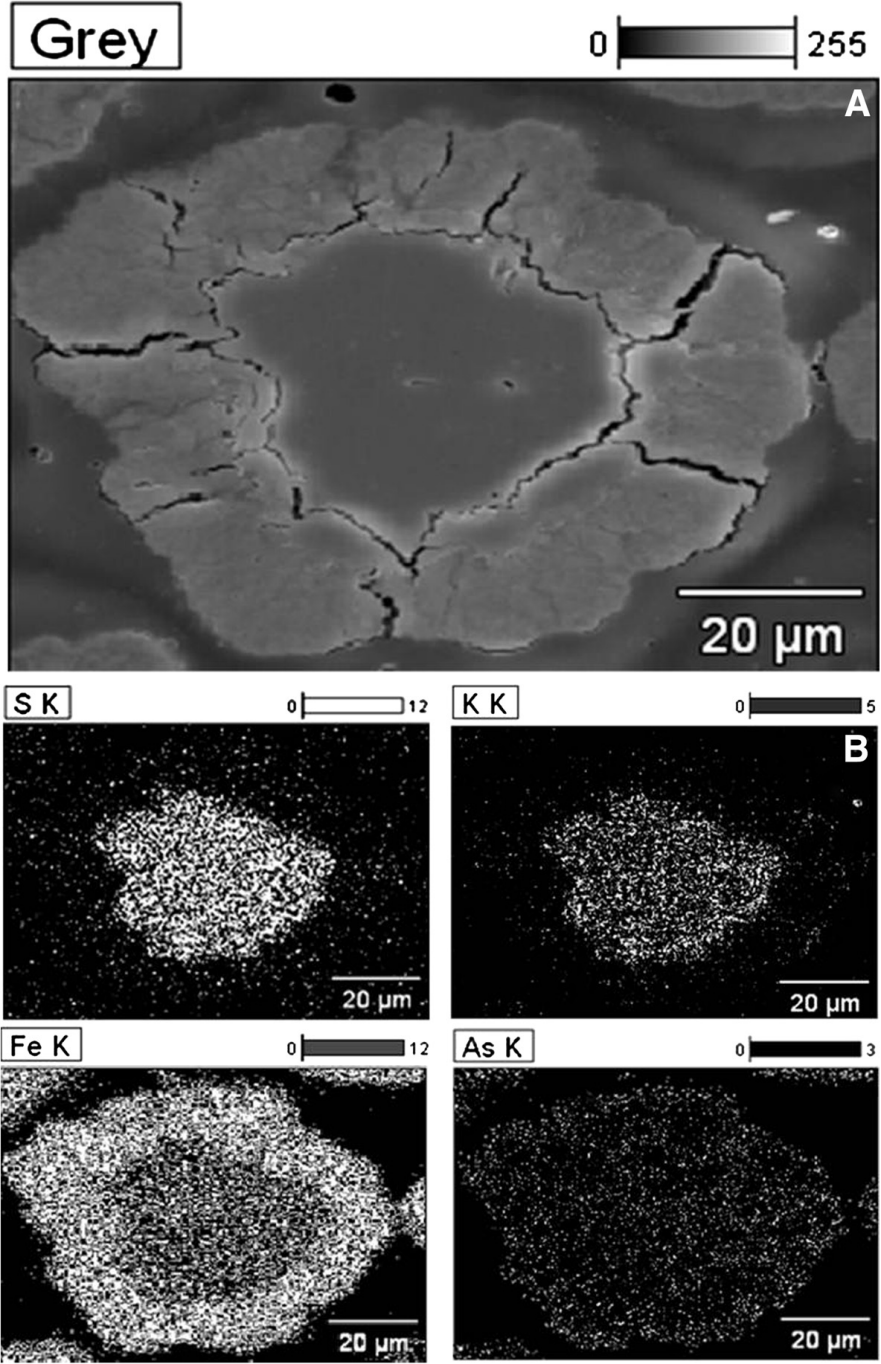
(A) Partially reacted particle and (B) X-ray map. Figure 5A shows a partially decomposed particle made of a gel halo, a reaction front and an unreacting core. Figure 5B shows the mapping performed on the partially decomposed particle. In the part showing the gel halo it can be observed how K and S have diffused from the particle into the solution, and are present only in the unreacting core. Fe and As are distributed throughout the particle. Previous studies have reported that the arsenic remains in the solids of the alkaline decomposition of arsenical natrojarosite [19].
